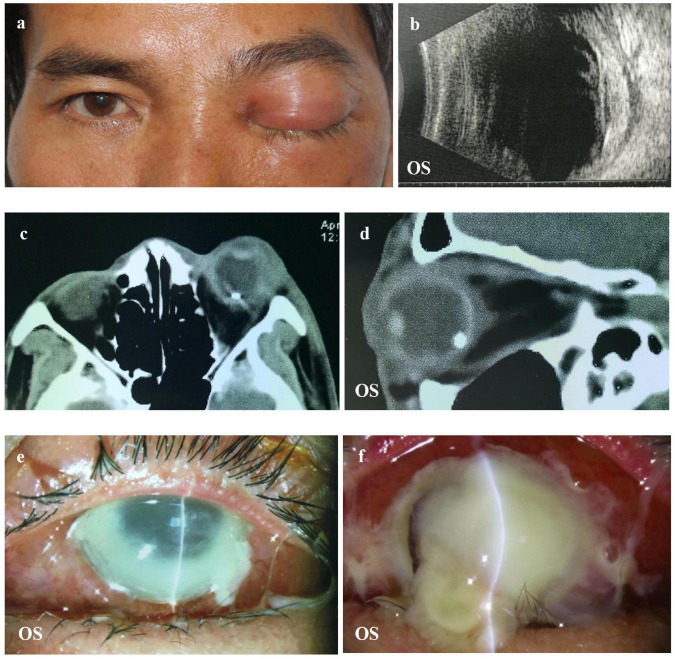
Representative image of a patient with OC. (a,b) The patient complained of vision loss and orbital pain 1 day after a foreign body splashed into the left eye. B-scan showed increased choroidal thickness. (c,d) CT showing inflammation of the orbital tissue and IOFB in the left eye. (e,f) Uncontrolled total corneal melting that required an evisceration. OC, orbital cellulitis; CT, computed tomography; IOFB, intraocular foreign bodies.
